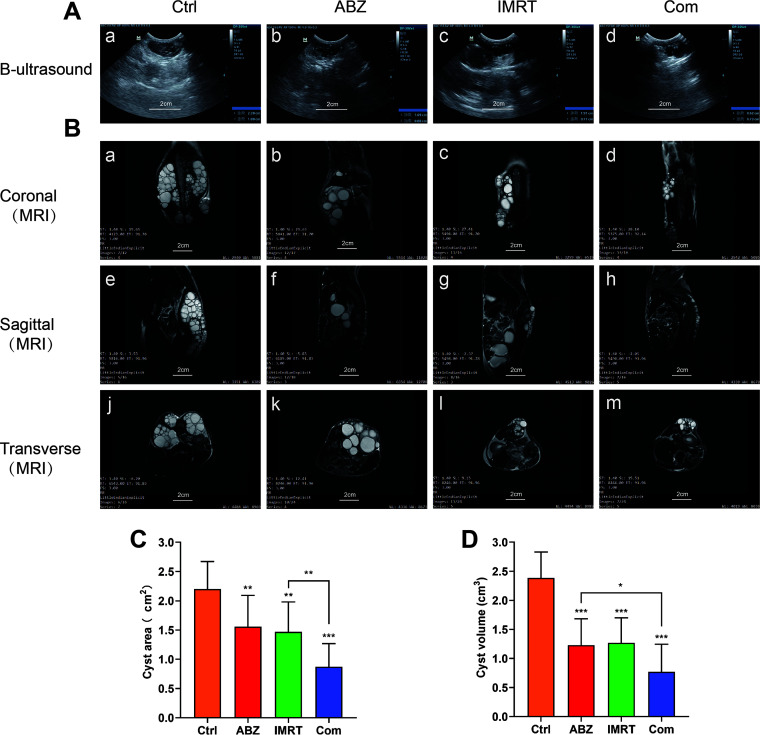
In vivo* effect of combination of ABZ-CS-MPs with IMRT in a spinal CE model. After 6 weeks of infection, MRI and B-ultrasound were used to dynamically detect the size and distribution of cysts in gerbils. (A) The location, shape, boundary, and internal echo of abnormal echo focus, blood flow signal, as well as maximum lesion diameter were recorded. Clear image was reserved to calculate the lesion area (cm^2^). Bars indicate 2 cm. (B) The maximum diameter of the vesicles was measured, and the cross-sectional area of the vesicles was calculated and compared. *, *P* < 0.001. (C) Images of sagittal, coronal plane, and transverse section were captured. MR diffusion software was used to analyze the images. Bars indicate 2 cm. (D) The maximum diameter was measured, and the volumes of cysts were calculated and compared. , *P* < 0.01; *, *P* < 0.001.
